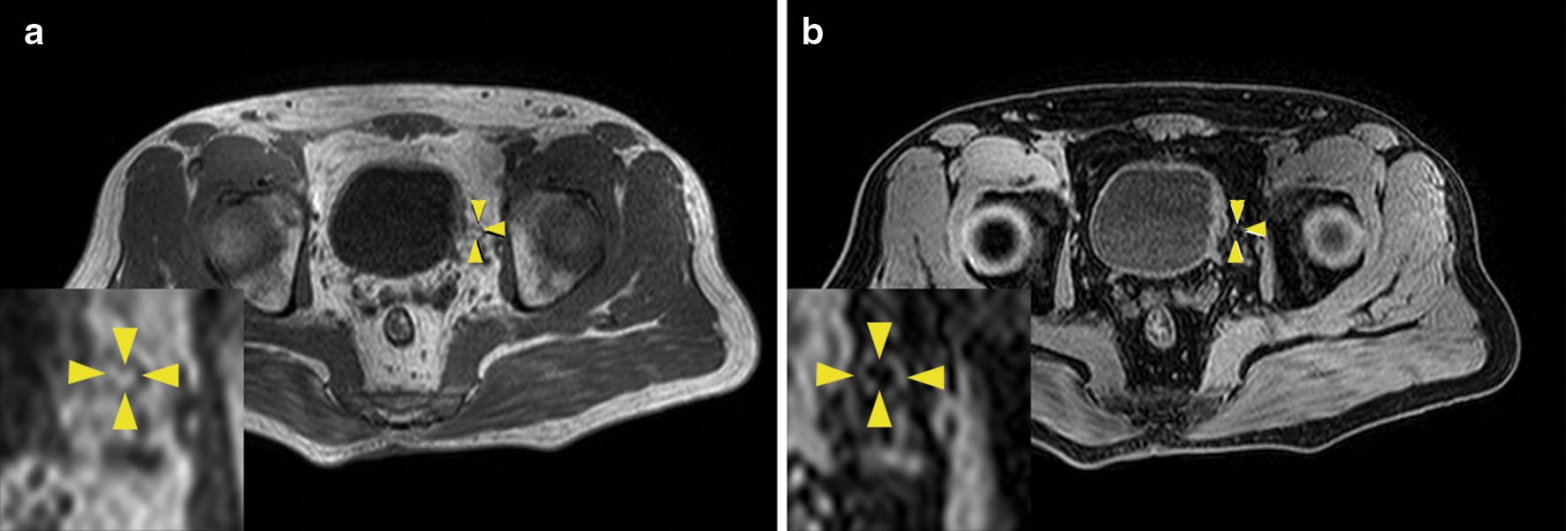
Pelvic magnetic resonance (MR) images of a 62-year-old man with bladder cancer (stage pT2b). a Transverse T1-weighted MR image shows a hyperintense lymph node with round shape and a short-axis diameter of about 6.0 mm in the left perivesical region. The fatty hilum shows high signal intensity on the image (yellow arrows). b In out-of-phase MR imaging, the fat signal was suppressed in the lymph node (yellow arrows). This lymph node with fatty hilum was proved to be benign in pathologic diagnosis
